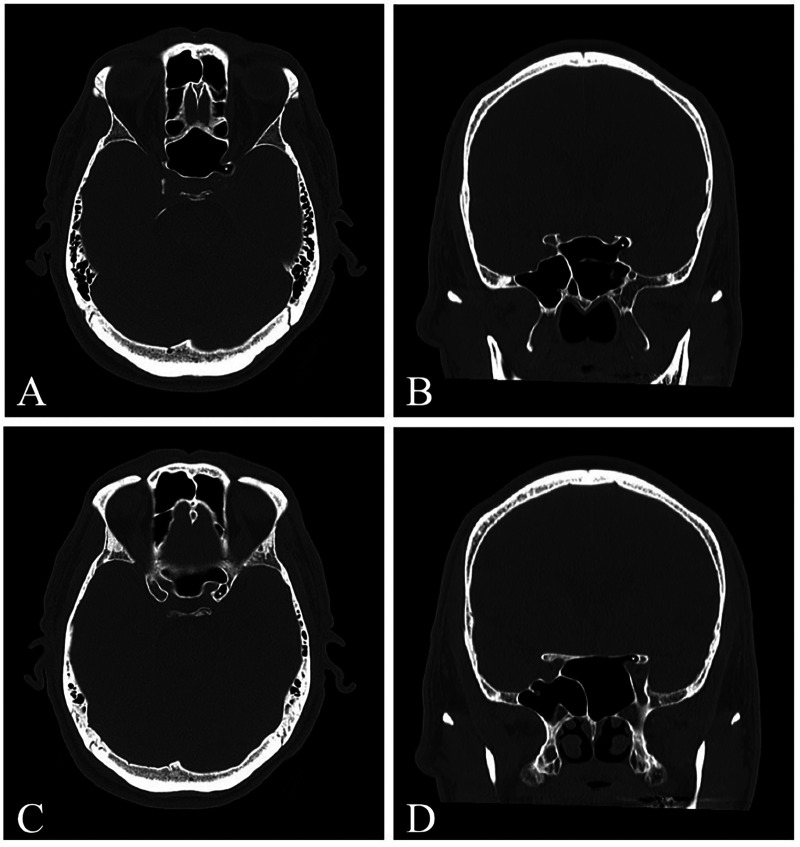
Type 4b anterior clinoid process (ACP) pneumatization (A,B) axial and coronal CT images demonstrating pneumatization through the optic strut (asterisks). (C,D) Axial and coronal CT images of the same patient demonstrating pneumatization through the planum sphenoidale (asterisks). Together, these findings represent combined optic strut and planum pneumatization with >50% ACP involvement (Type 4b).
